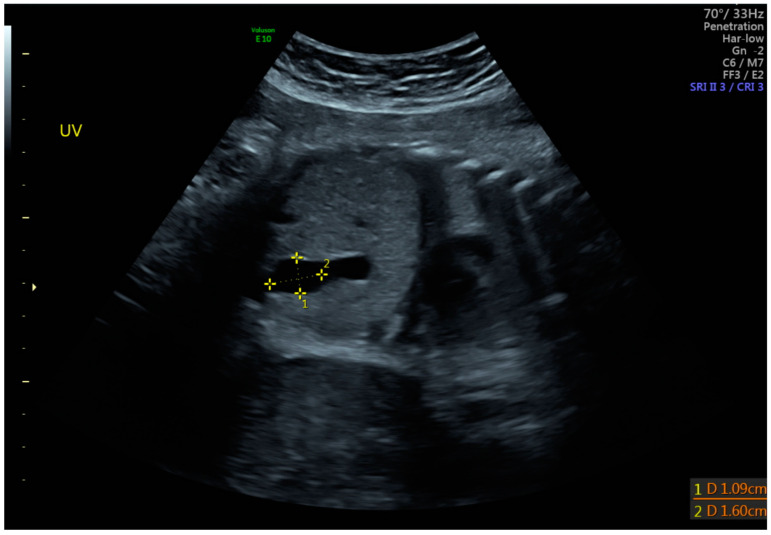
Ultrasound demonstrating measurements of an umbilical vein varix with gray scale.
